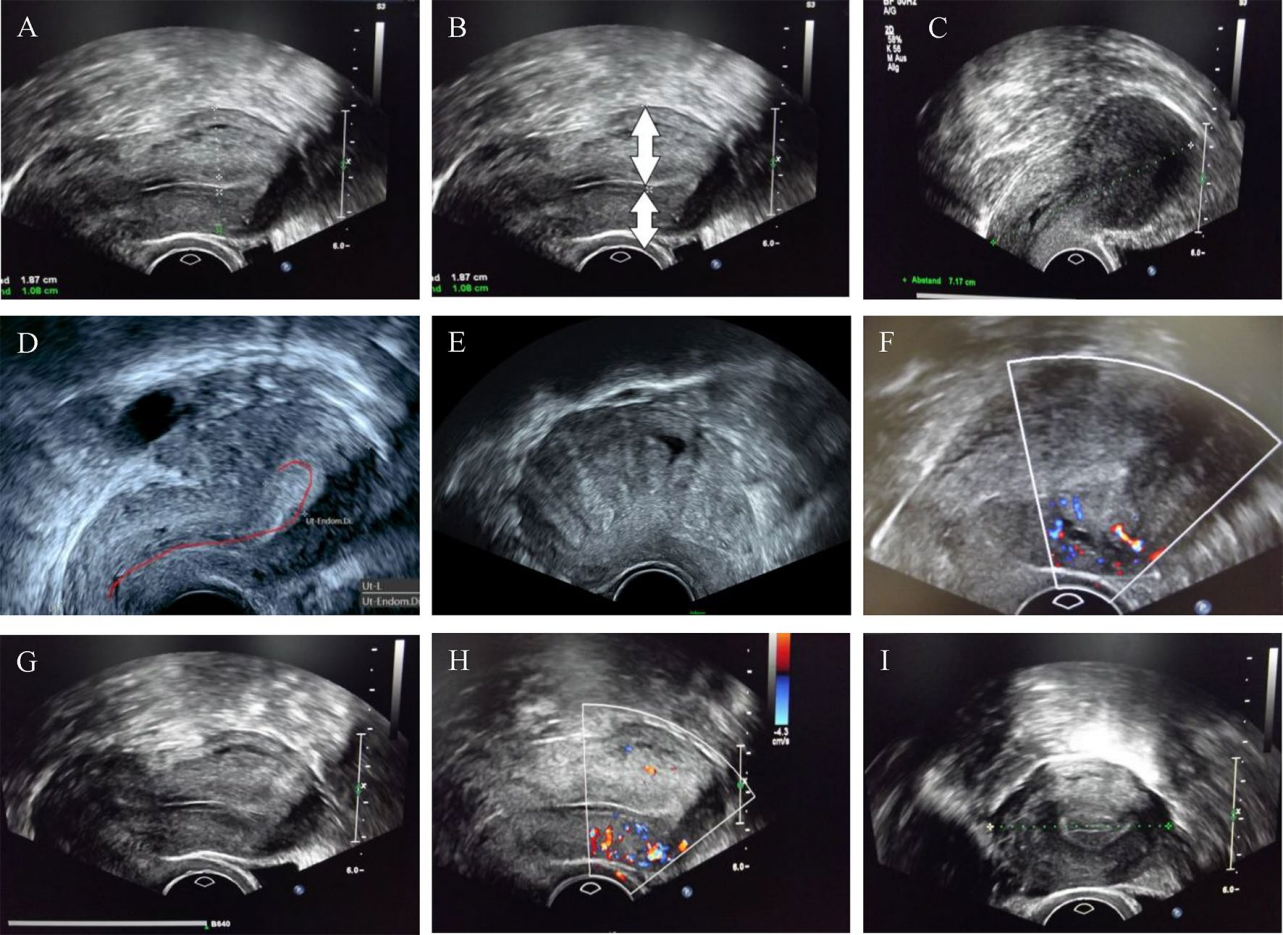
Adenomyosis-specific sonographic markers. A Asymmetric walls of uterus. B Asymmetric uterus walls. Marked for optimal visualization. C A blurred junctional zone of the endometrium and myometrium. D A question mark form of the uterus. E “Rainforest-Phenomen” of the uterus. F Intramural lacunae of the myometrium. G Heterogenic echogenicity of the myometrium. H Diffuse vascularisation of the myometrium. I Enlarged globular fundus uteri. All images were taken in our clinic
